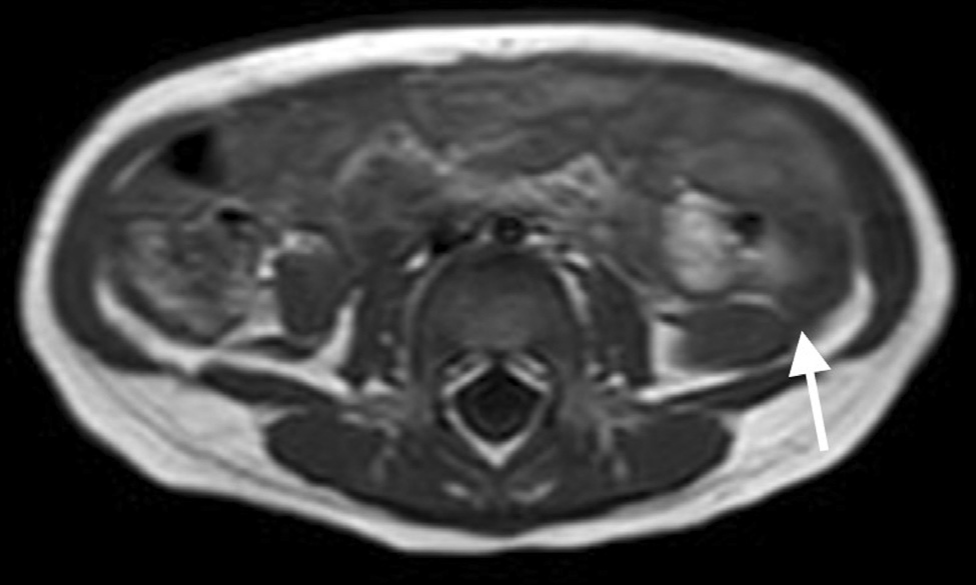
Splenic cord-like structure on magnetic resonance imaging (arrow).
